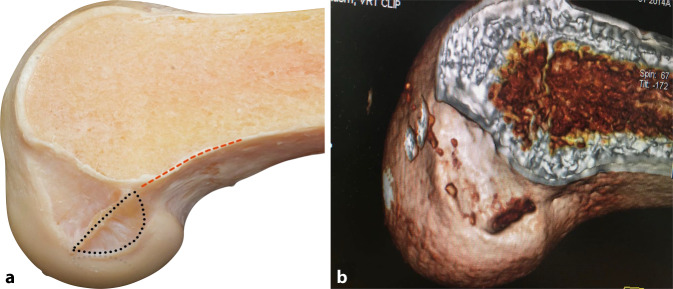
a Anatomisches Präparat mit direkter VKB-Insertion femoral in Verlängerung der dorsalen Kortikalis *(rot strichlierte Linie*) und indirektem Insertionsareal (*schwarz punktiert*). b Postoperative 3D-CT-Rekonstruktion einer VKB-Rekonstruktion mit flachem femoralen Kanal im anatomischen Insertionsareal
